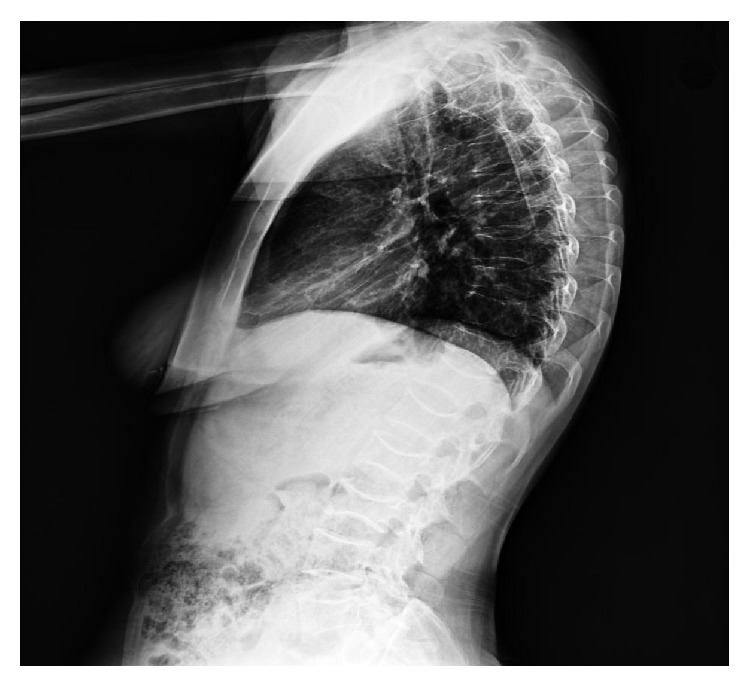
Lateral thoracolumbar X-ray; loss of height in vertebrae and biconcave appearance in vertebrae.
